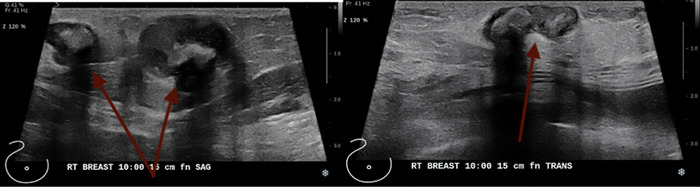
Right breast ultrasound demonstrates multiple abscesses at the 10 o’clock position 15 cm from the nipple.
